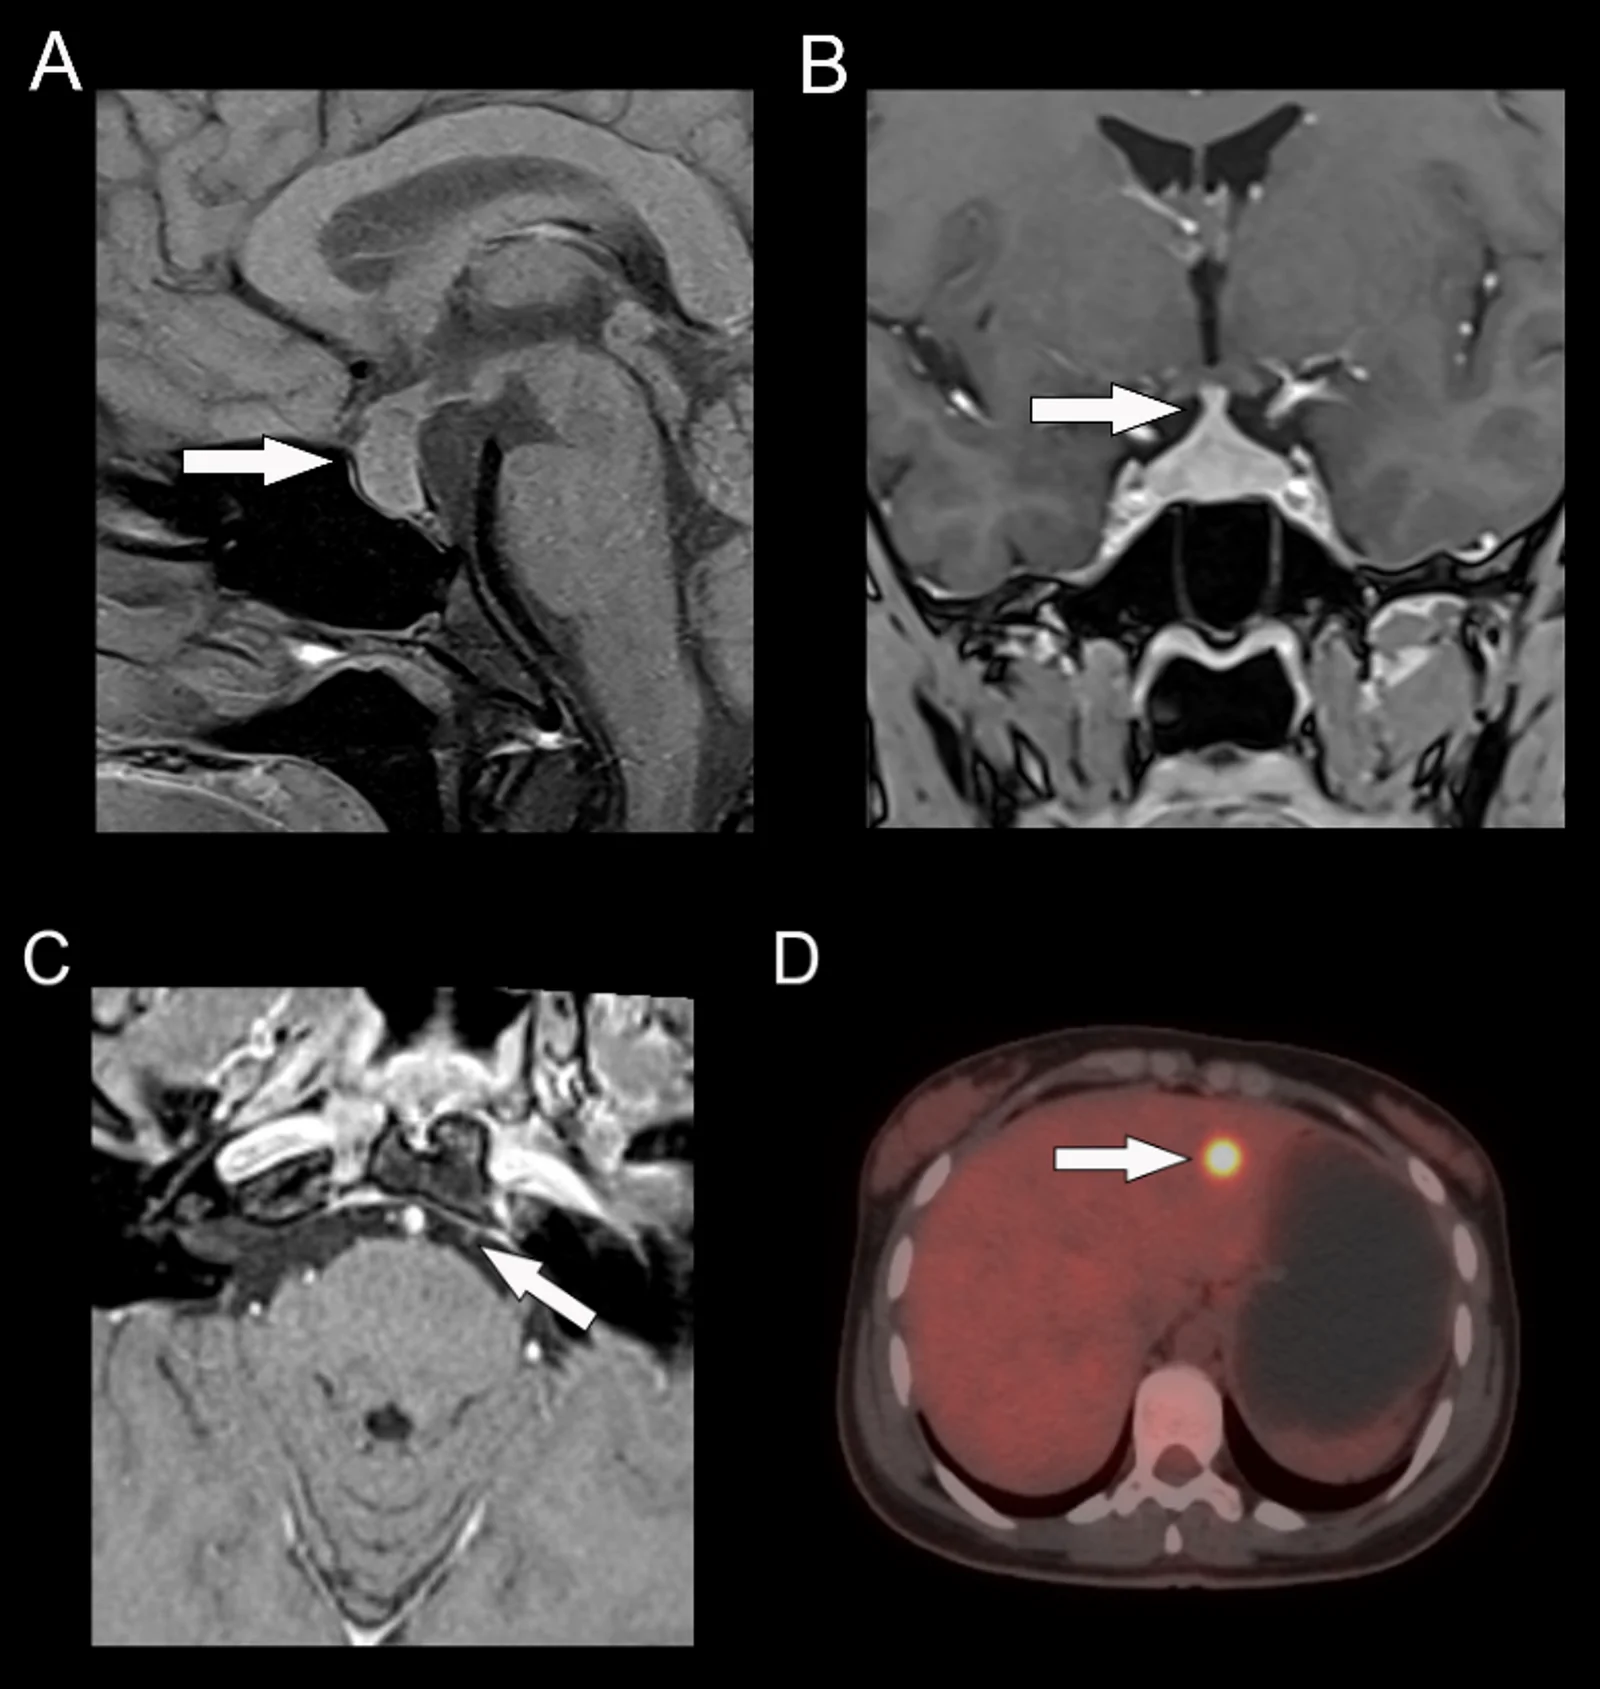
Characteristic imaging findings in a patient with hypophysitis due to neurosarcoidosis. Sagittal T1-weighted image (A) demonstrates a sellar lesion encompassing the pituitary gland (arrow), with absence of the normal T1 hyperintense signal of the posterior pituitary (neurohypophysis), consistent with loss of the posterior pituitary bright spot. Coronal postcontrast T1-weighted image (B) shows heterogeneous enhancement of the pituitary lesion (with a major axis of 20 mm), associated with pituitary stalk thickening (arrow). Axial postcontrast T1- weighted image (C) discloses abnormal enhancement of the distal cisternal portion of the left abducens nerve (arrow). FDG PET-CT image (D) reveals a hypermetabolic nodular lesion in the left hepatic lobe (arrow).
